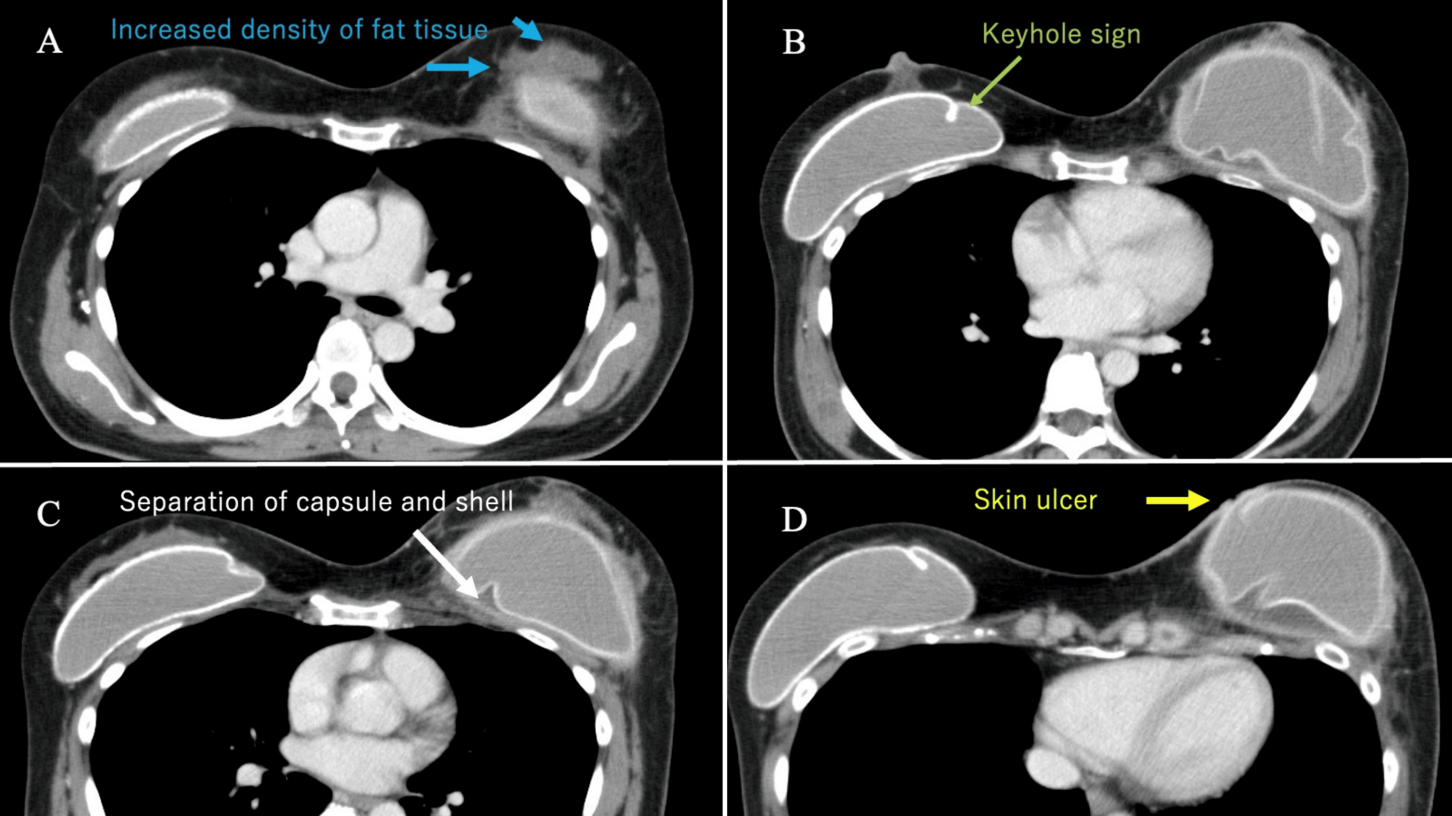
Contrast-enhanced CT findings, showing (A) increased density of adipose tissue around the SBI (blue arrows), (B) keyhole sign (green arrows) within both SBIs, (C) separation of capsule and shell (white arrows), and (D) skin ulcers (yellow arrows) CT: computed tomography; SBI: silicone breast implant
